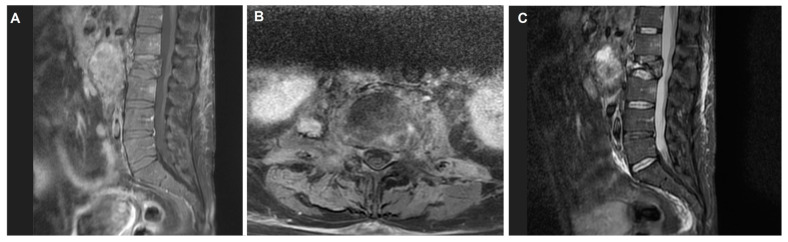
Preoperative lumbar spine MRI showing heterologous lesions at the L1 vertebra with collapse of the L2 vertebral body. (A) Sagittal T1-weighted image with contrast enhancement reveals a hypointense lesion in L2 with marked contrast uptake. (B) Axial T1-weighted image with contrast highlights irregular enhancement in the L2 vertebra extending into prevertebral structures. (C) Sagittal T2-weighted image shows a hyperintense lesion in L2 vertebral collapse with associated signal changes, indicating edema or bone marrow involvement.
